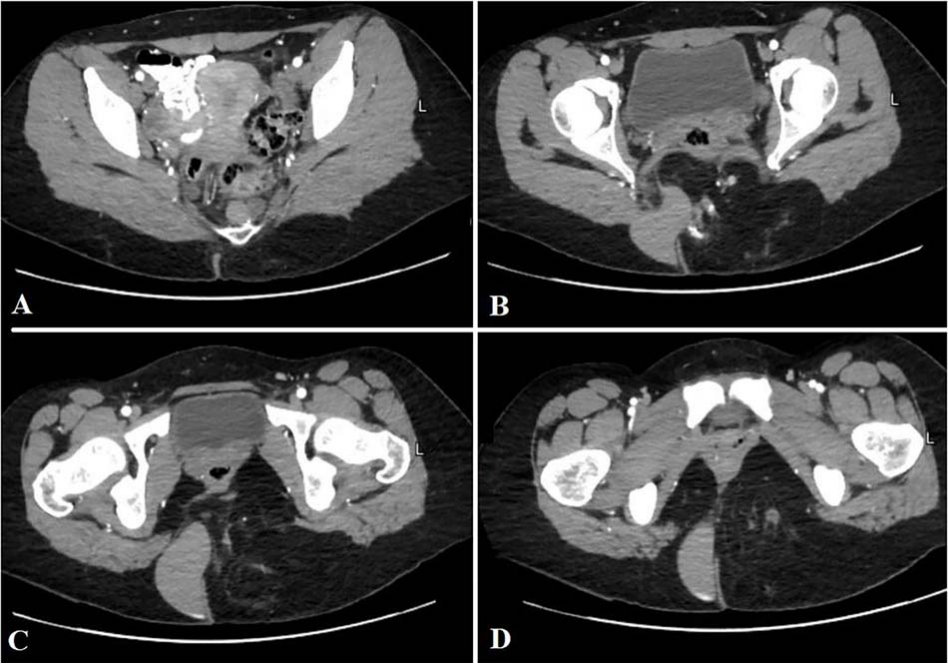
Selected axial CT cuts of the pelvis with oral and IV contrast (Arterial phase) from above downward (A–D), showing the mentioned gluteal mass measuring about 9 × 15 × 0.5 cm^3^. The boundaries of the lesion were not clearly defined and the dimensions were assumed based on its mass effect on the adjacent structures. The lesion consisted of mixed tissues (fat predominantly, fluid and calcifications). The fluid portion appeared on the right aspect of the gluteal region with high density, indicating mucinous/proteinaceous content. Extensions into the ischio-rectal fossa are noted with minimal pre-coccygeal components seen as well.
